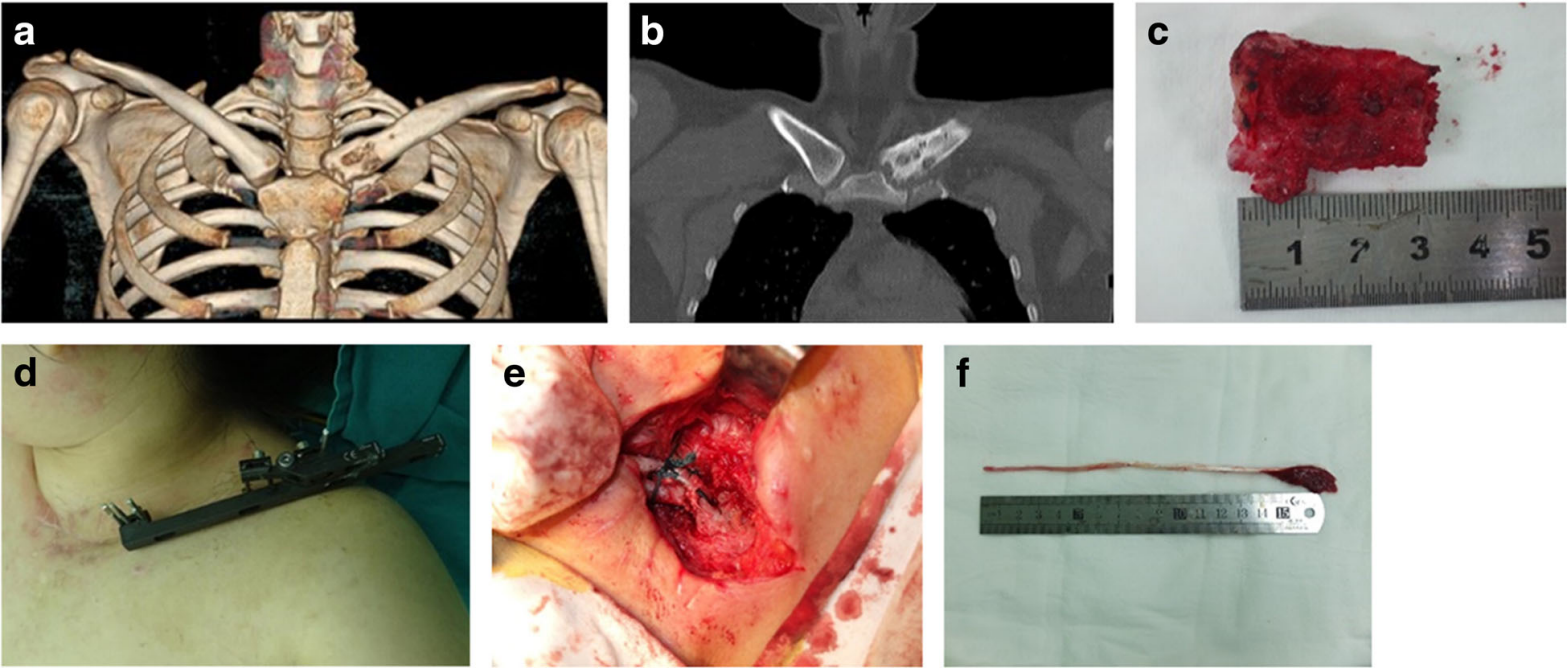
Surgical procedures of the three-stage treatment including debridement, bone transportation, and tendon autografting. a–c The first stage: resection and debridement. A preoperative CT image shows bone destruction with surrounding inflammation. d The second stage: bone transportation. The clavicle length is increased using distraction osteogenesis. e, f The third stage: tendon grafting. An autologous palmaris tendon graft is placed in a figure-of-eight fashion for restoring the stability of the SCJ
